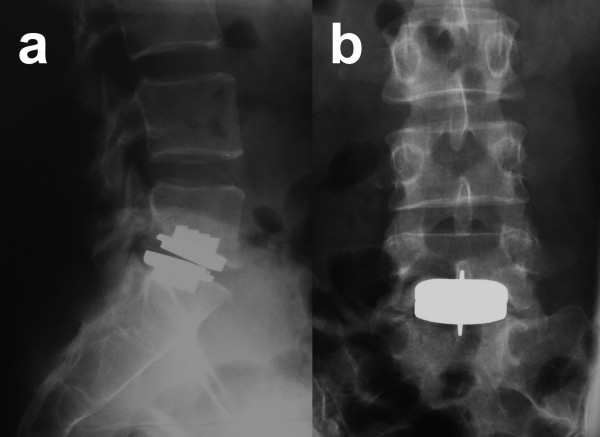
A 42-year old female patient with degenerative disc disease received implantation of an artificial disc at L5-S1 (Prodisc, Synthes). Postoperative acute bilateral S1-pain without motor deficit could be explained by posterior dislocation of the inferior plate and a subsidence of the cranial plate of the disc prosthesis (a, b). This could clearly be visualised on plain radiographs. No dislocation of the PE inlay occurred which can be seen in the radiographic marker, being still in place. This patient was treated with a conversion into anterior fusion at L5-S1 with pain relief at the 1 year follow-up.
